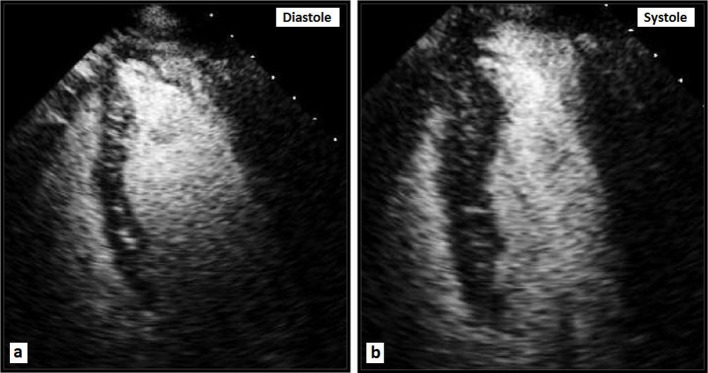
Apical four-chamber TTE views with Definity contrast demonstrating a large apical diverticulum.
